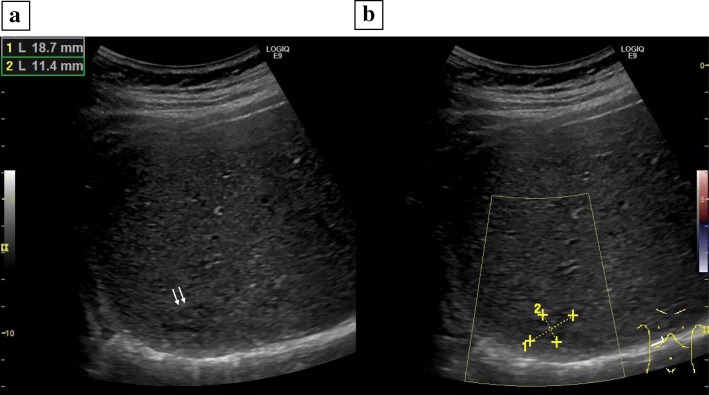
Findings of abdominal ultrasonography. a a 18.7 × 11.4 mm heterogeneous iso- and hypoechoic mass which displayed irregular shape and indistinct margin and included hyperechoic spots in segment 7 of the liver with the B-mode ultrasonography (white arrows), b The liver mass showed no flow signals in the color doppler mode
